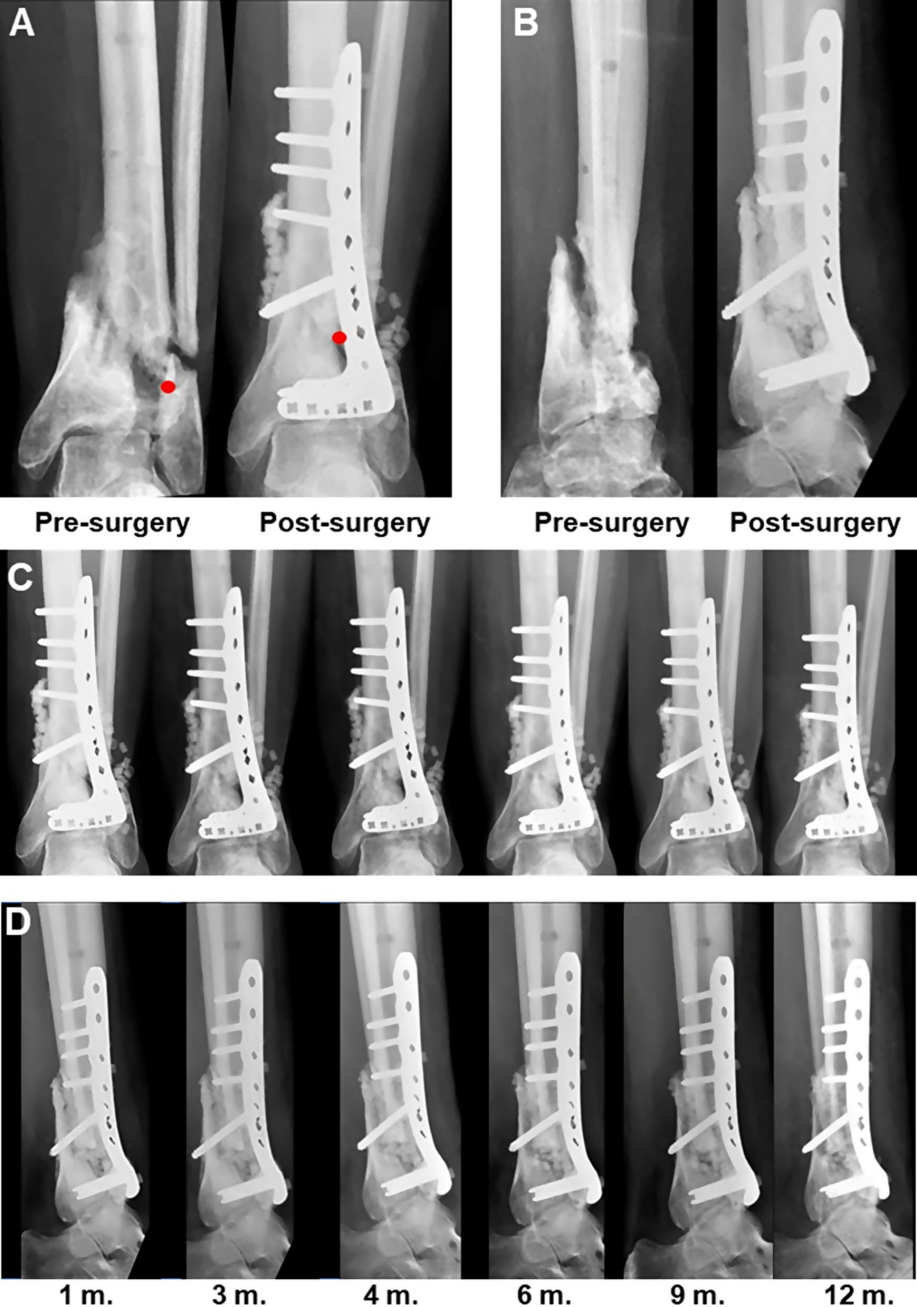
Example of use of allogeneic AT-MSC in a tibia nonunion (patient 1-14-10). X-ray anteroposterior (A and C) and lateral projections (B and D). A and B show fracture before and after surgery; C and D show the follow up until month 12. The 54-year-old female patient initially presented an open tibial pilon fracture treated with external fixation and minimal osteosynthesis with Kirschner wires. Sepsis was confirmed three weeks later, which was treated with debridement, circumferential fixation, and antibiotics. After three months the external fixation was withdrawn and replaced by a cast, and subsequently by a removable orthosis without load. The fracture evolved into a nonunion. Thirteen months after the initial fracture, and after ruling out a septic process, curettage of the wound was conducted. Once an acceptable anatomical realignment had been achieved, a large space lost bone was observed inside the metaphysis; the contact area between segments was very limited and only on the internal slope of the tibia. Stability was achieved with a lag screw and an anterolateral distal tibial plate overlying it. An allogenic biograft consisting of 98 monetites was applied, with a total dose of 39 × 10^6^ AT-MSC. The patient presented a good postoperative course, without inflammatory signs, and was discharged from the hospital after 4 days without immobilization. Healing was verified at 6 months, with gait being painless and presenting only limited joint balance in dorsiflexion (5º)
